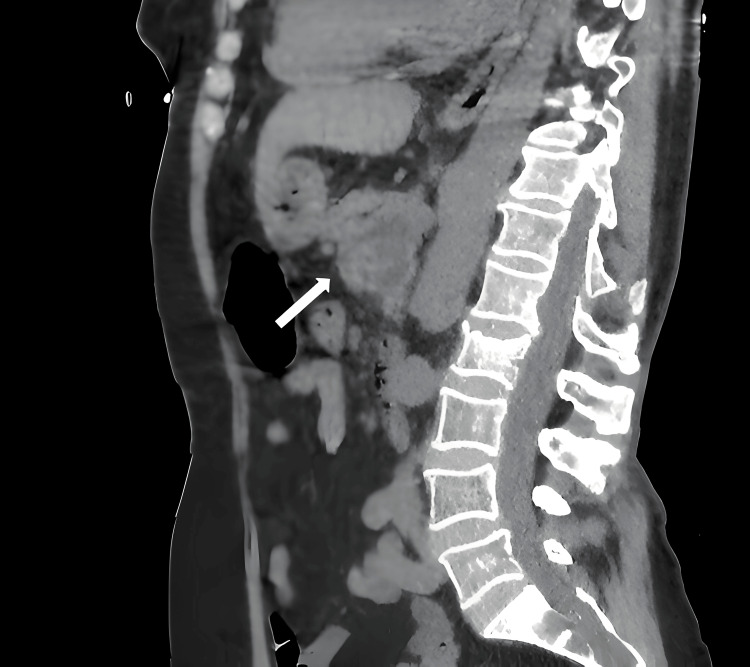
Pancreatic hydatid cyst (indicated by the white arrow): CT image in the sagittal section (personal case study).
